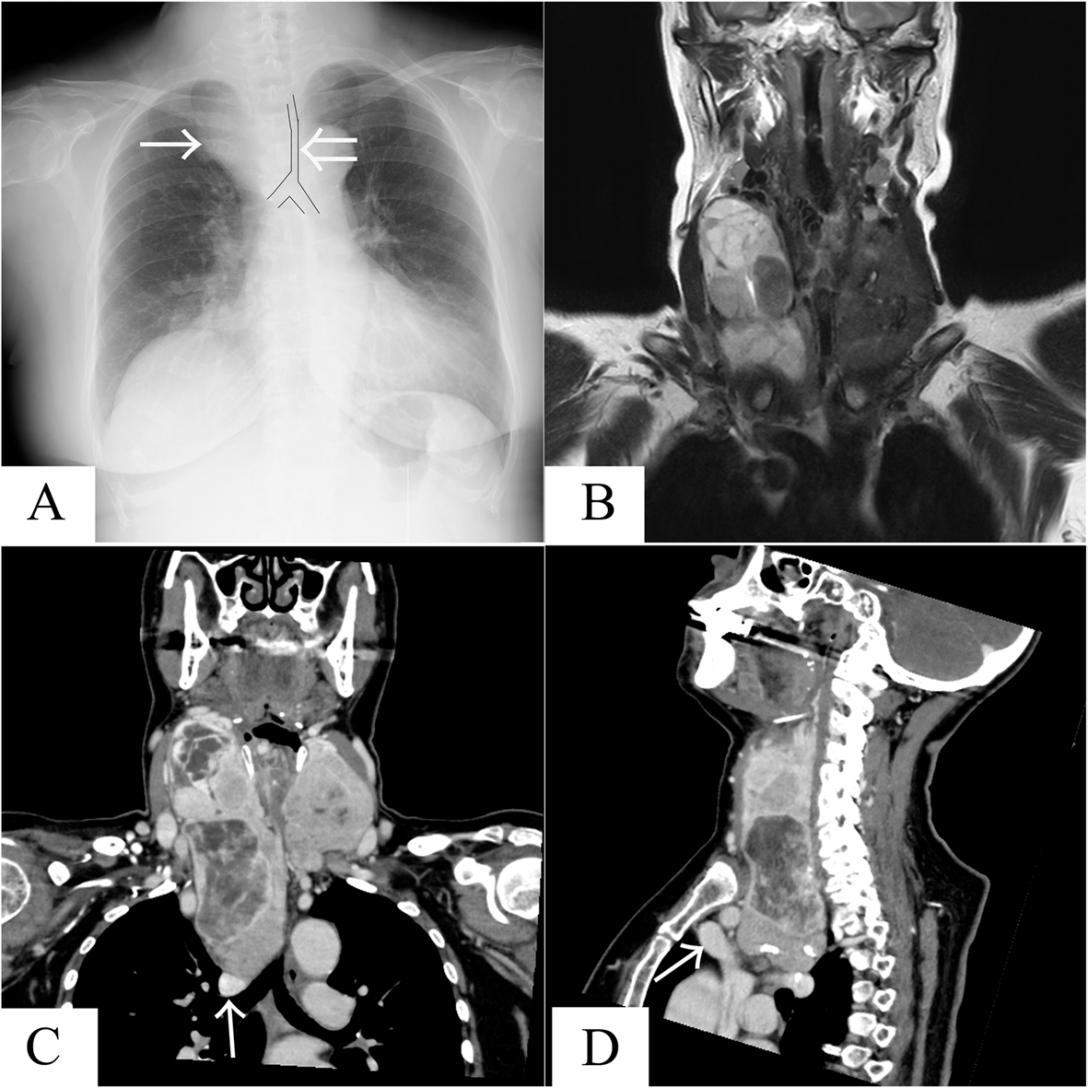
Image findings at admission. A Chest X-ray revealed the tracheal stenosis (large arrow) and deviation to the left side (small arrow). B Enhanced magnetic resonance imaging of the neck and chest showed a giant goiter with heterogeneous contrast growing in both thyroid lobes, and the trachea was pressed by the goiter and had become narrowed. C Coronal section of enhanced computed tomography of the neck and chest showed that caudal edge of the right goiter extended along the trachea just cranial to the azygos vein (small arrow) with no invasion. D A sagittal section of enhanced computed tomography showed that the goiter was not invading the right brachiocephalic vein (small arrow)
